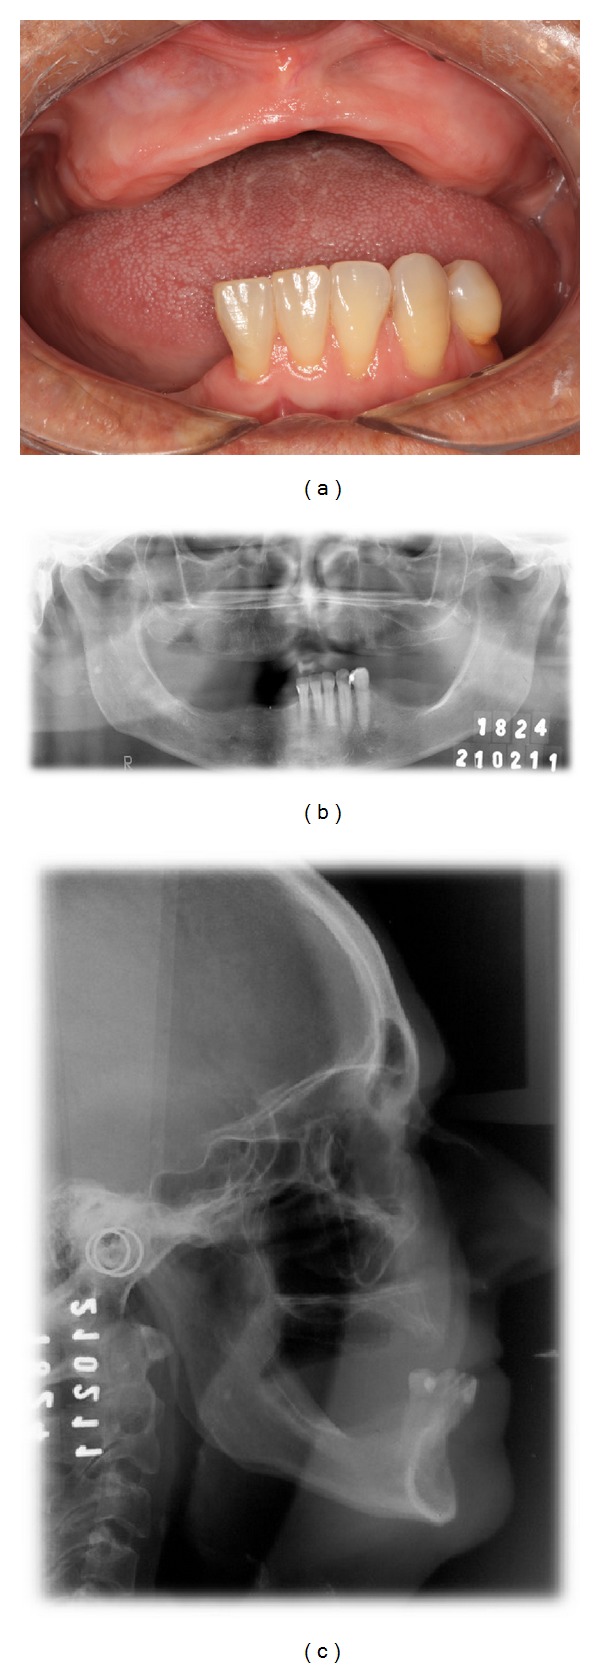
(a) Clinical exam: edentulous maxillary and partially edentulous mandible. (b) Radiographic exam: panoramic radiography. Mandibular height favoring dental installation implants. (c) Radiographic exam: teleradiography. Favorable maxillomandibular relationship to the installation of dental implants and prosthetic rehabilitation treatment.
